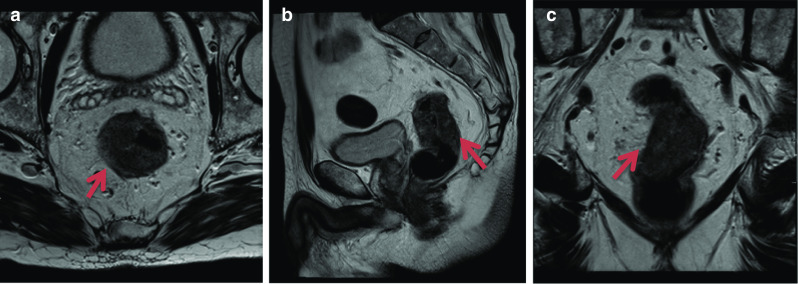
Rectal adenocarcinoma* Axial, sagittal and coronal T2-weighted images showing a semi-annular mass (red arrow), posterior to the prostate and within the rectum which is T2 hyperintense to gluteal muscle and extending from 3 to 1 o’clock causing significant luminal narrowing. Colonoscopy and biopsy proved rectal adenocarcinoma
